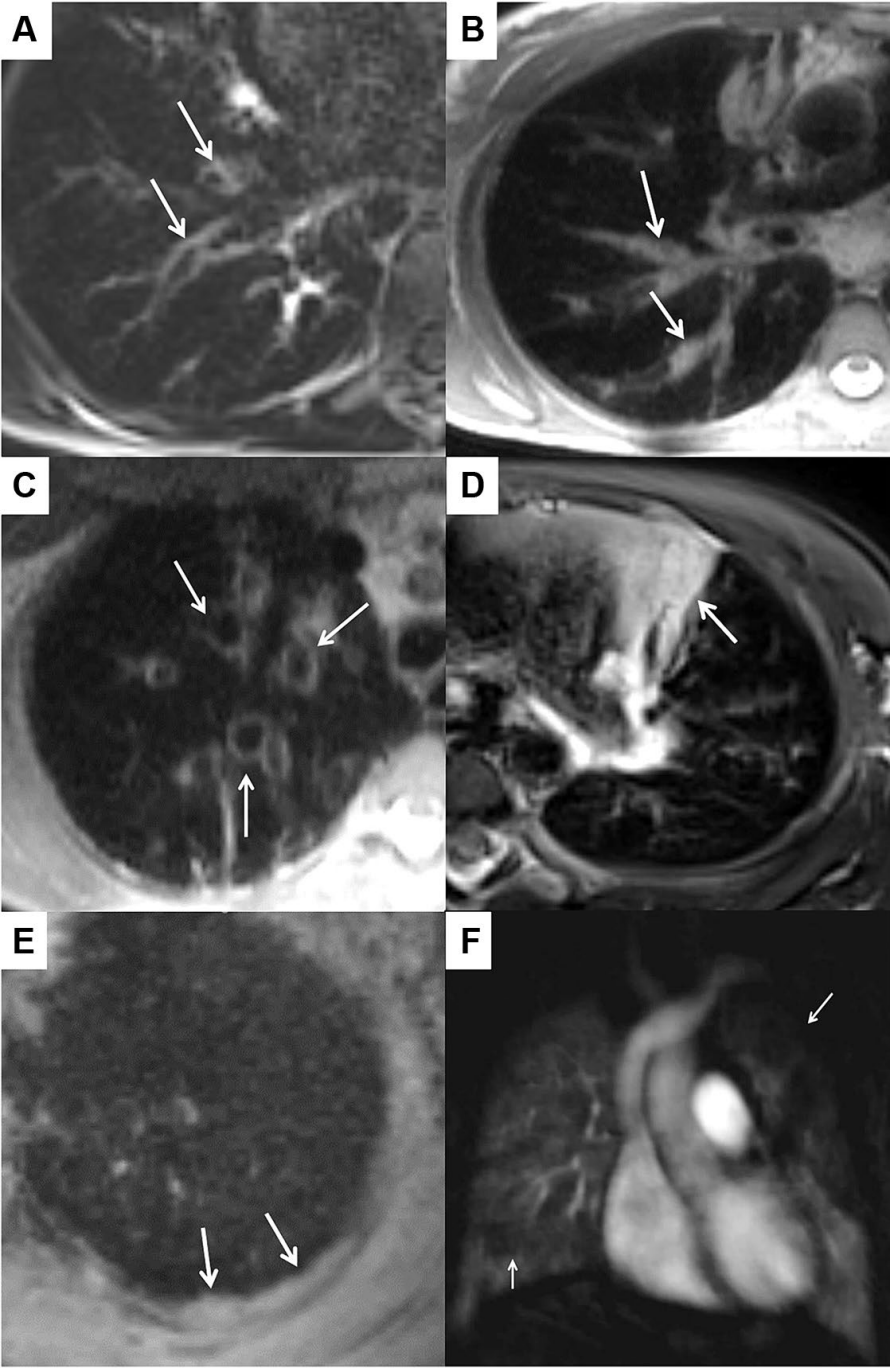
CF-related MRI findings representing the subscores of the morpho-functional chest MRI score. (A–E) Show image sections of a high-resolution T2-weighted sequence acquired in transversal plane. The arrows indicate bronchiectasis/wall thickening (A), mucus plugging (B), sacculations (C), consolidation (D) and an inflammatory pleural thickening (E), which is part of the score as a “special finding.” (F) Shows a post-processed subtraction image from the contrast-enhanced perfusion MRI acquired in coronal plane. The arrows mark a large perfusion deficit in the left upper lobe and a small perfusion deficit in the middle lobe. By definition, each subscore in each lobe is assessed individually and 0–2 points are scored per lobe depending on whether the corresponding pathology is not present (0), involves less than 50% (1) or more than 50% (2) of the lobe.
